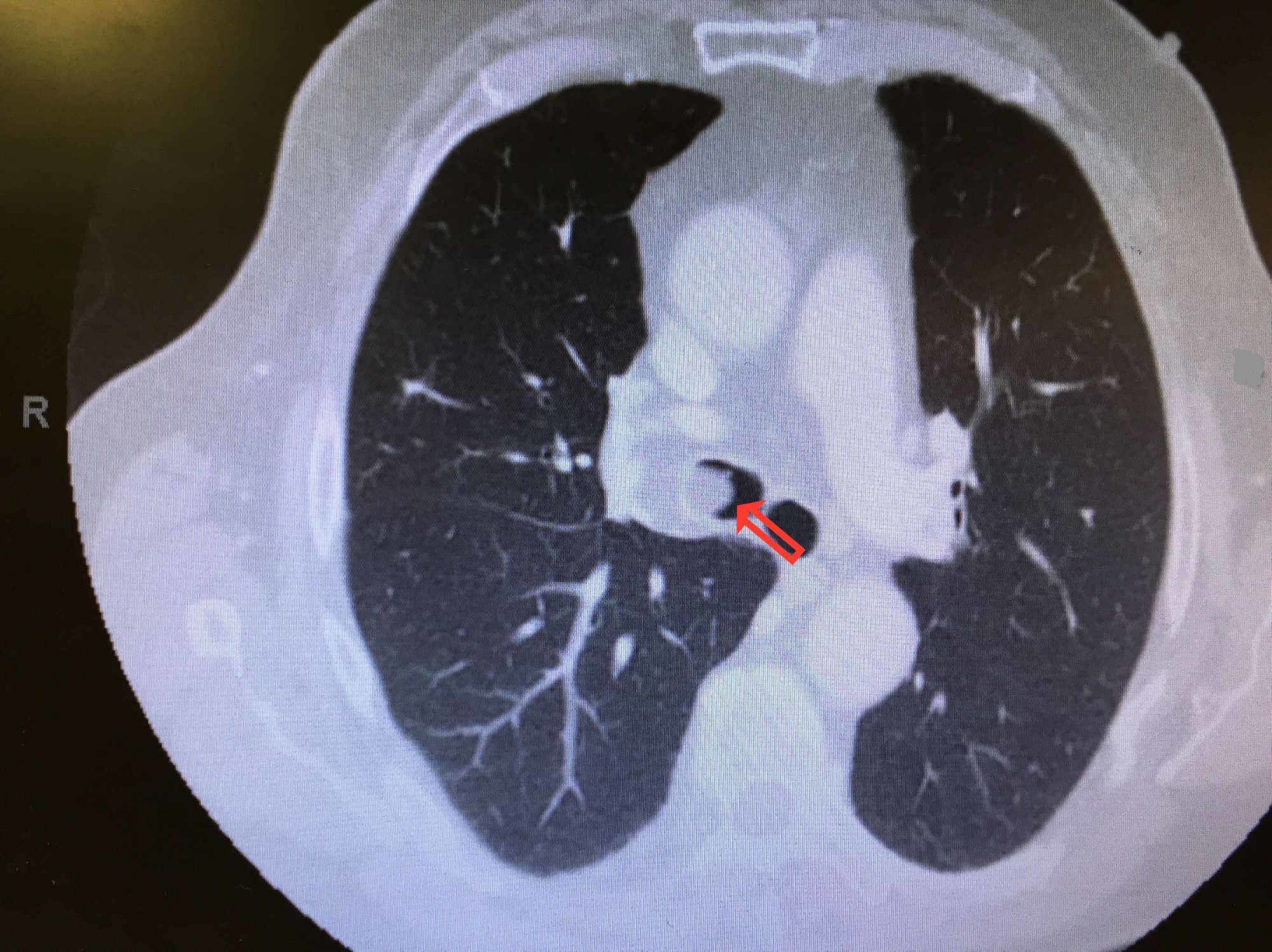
Computed tomography (CT) of the chest showing the obstructing lesion in the right mainstem bronchus
